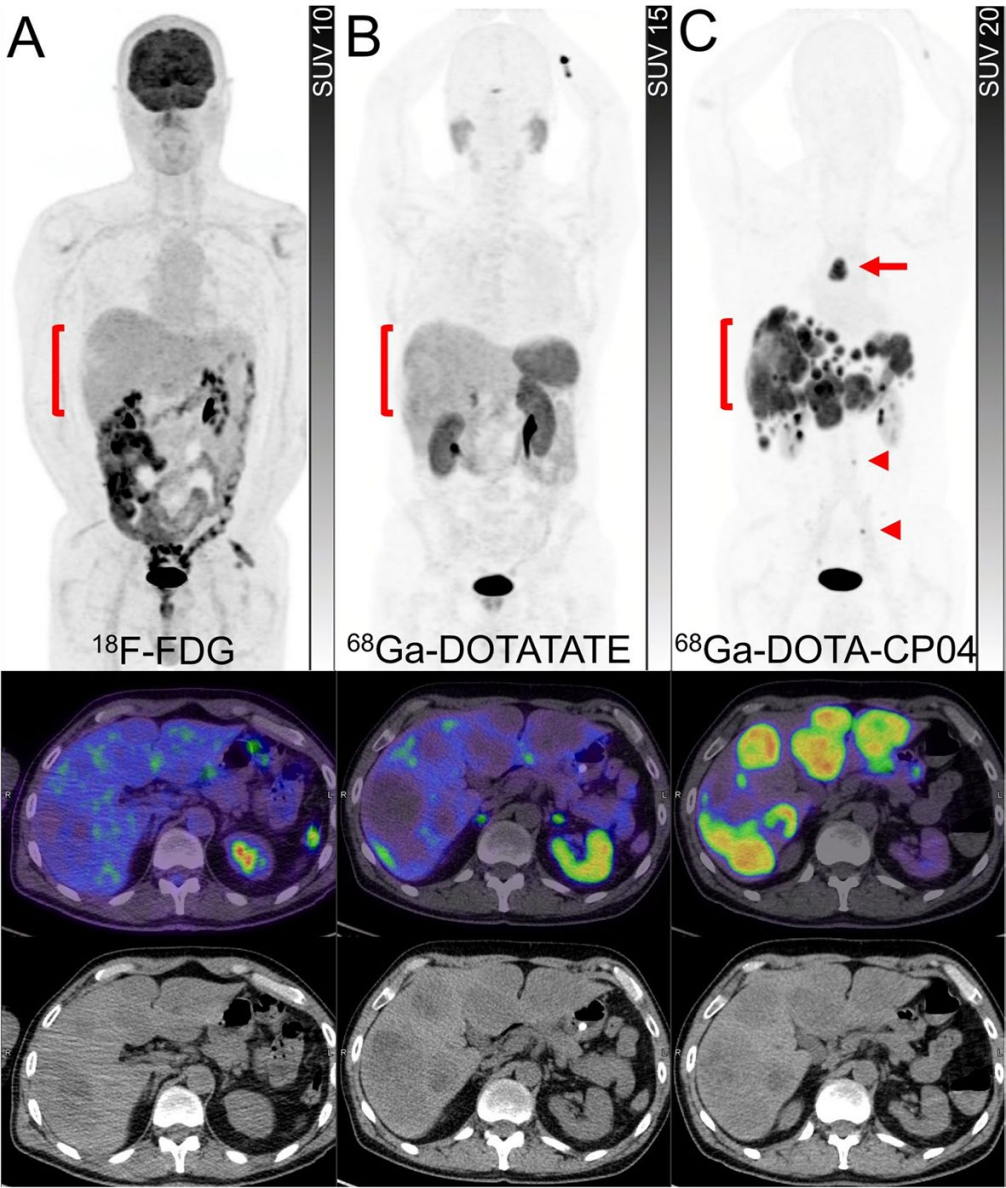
A patient with a progressive bronchial carcinoid tumour was referred for suitability for ^177^Lu-DOTATATE therapy. ^18^F-FDG PET, PET/CT and CT (A) showed non-avid hepatic metastases (brackets). ^68^Ga-DOTATATE PET, PET/CT and CT (B) demonstrated minimal tracer uptake in the periphery of hepatic metastases (brackets), indicating a lack of suitability for ^177^Lu-DOTATATE therapy. ^68^G-DOTA-CP04 (CCK-2 peptide receptor analog) PET, PET/CT and CT (C) showed intense uptake in all hepatic metastases (brackets) as well as a mediastinal nodal (arrow) and small bone metastases (arrowheads)
